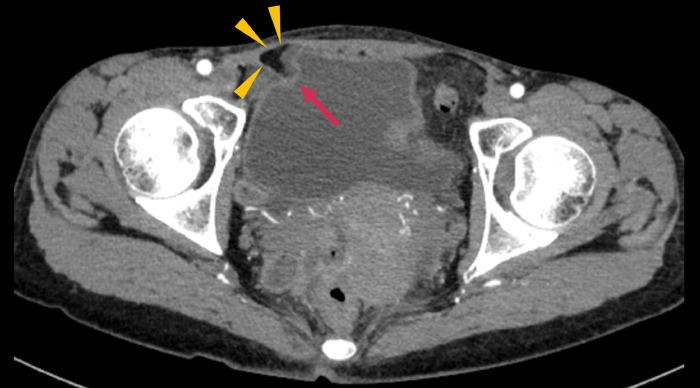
Findings from CT. CT revealed curved fatty tissue (arrowheads) protruding toward the bladder (arrow) from the right inguinal region.
